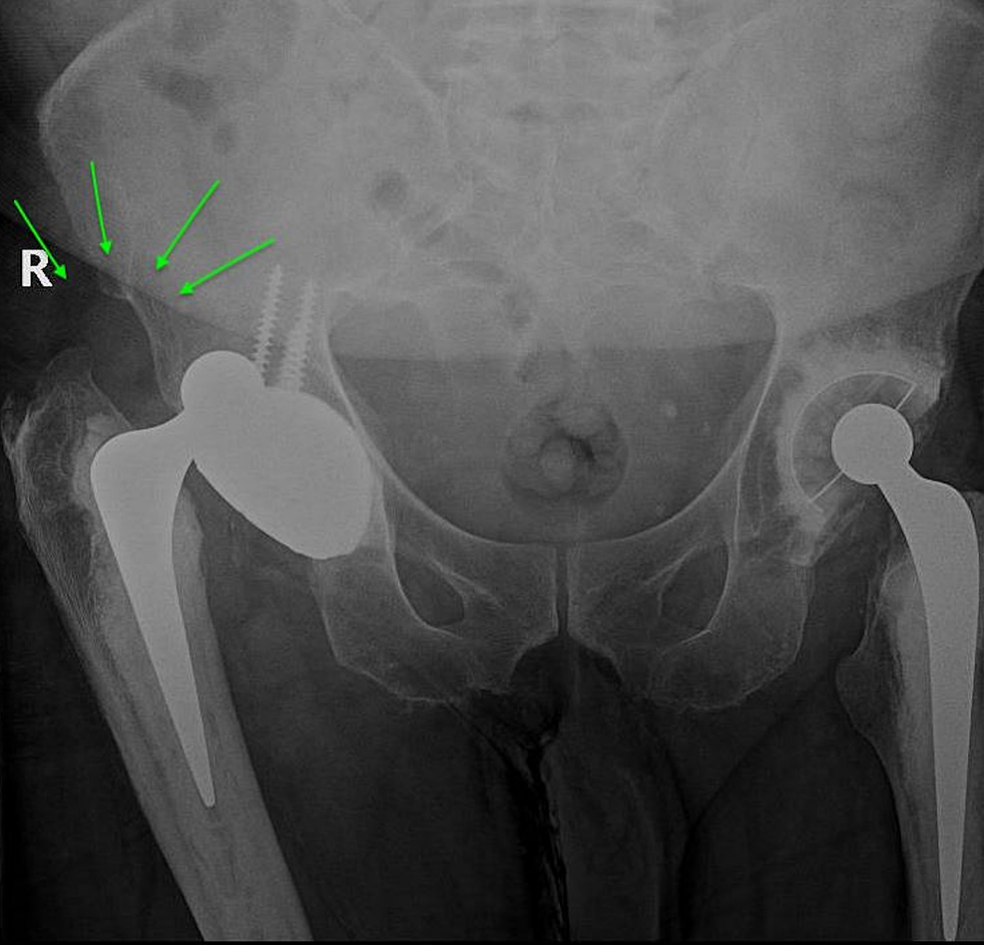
Anteroposterior pelvic X-ray showing intraprosthetic dislocation. The dislocated polyethylene liner is demonstrated by green arrows and appears as “the bubble sign.”
